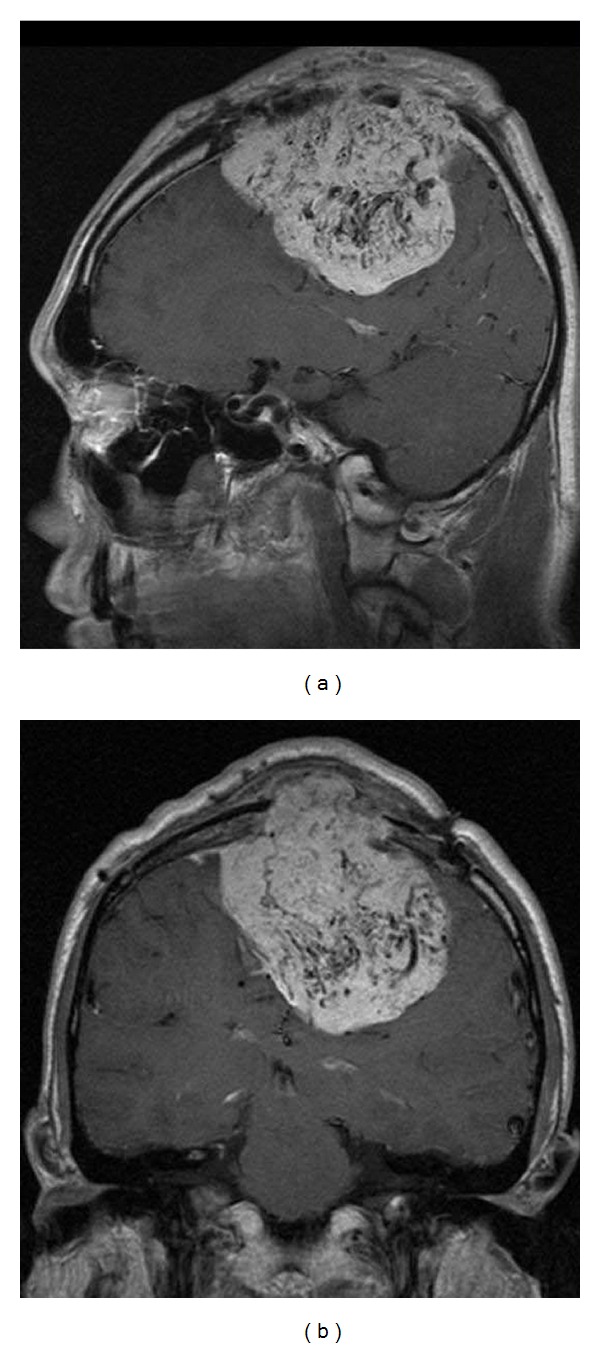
Preoperative sagittal (a) and coronal (b) T1-weighted magnetic resonance (MR) images with contrast medium showing the huge parasagittal meningioma. Note the dense flow void networks in the tumor.
